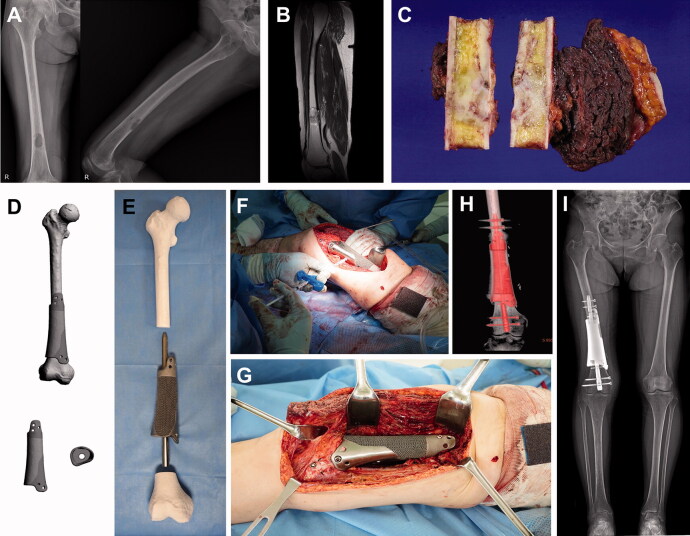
Femoral reconstruction. Images of patient #8. (A) Preoperative plain radiographs and (B) a T2-weighted MR image showing undifferentiated pleomorphic sarcoma of the bone arising from the distal femoral shaft. (C) Resected bone tumor as planned. (D) Graphical designs of the 3D-printed implant and (E) photograph of the 3D-printed implant and intramedullary nail to be used. (F) Intraoperative photograph showing cement injection through a premade hole. (G) Intraoperative photograph, (H) postoperative CT reconstruction image, and (I) teleradiogram showing femoral reconstruction.
